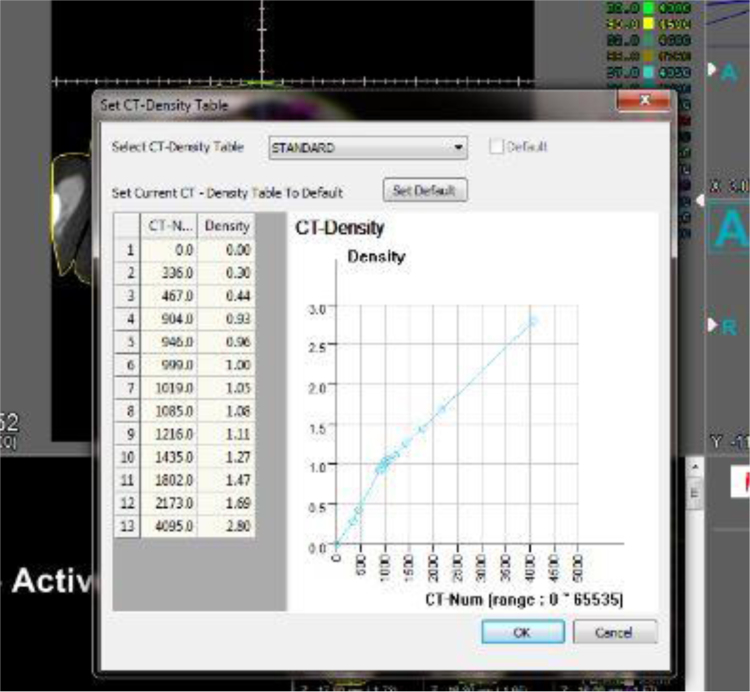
Mentioned transform chart for transformation CT-Number to density in CorePLAN software.
